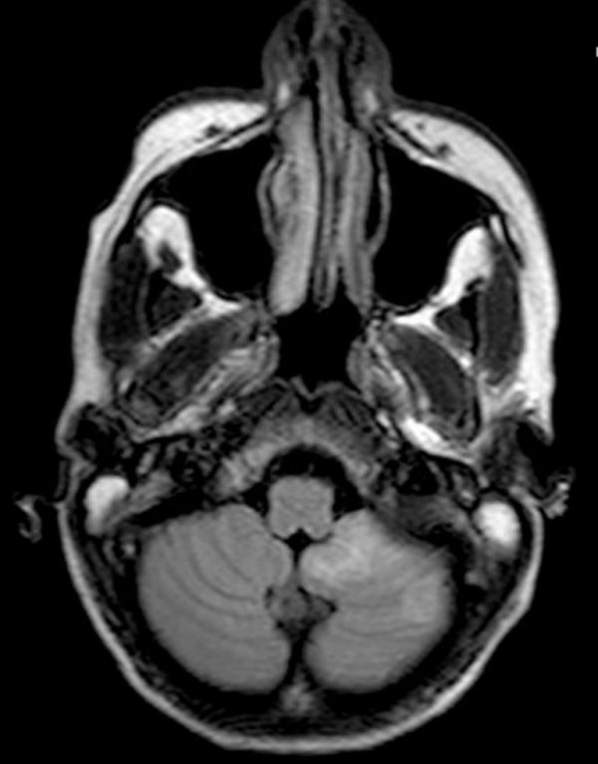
Brain magnetic resonance imaging (MRI) revealing left side cerebellar abscesses.
